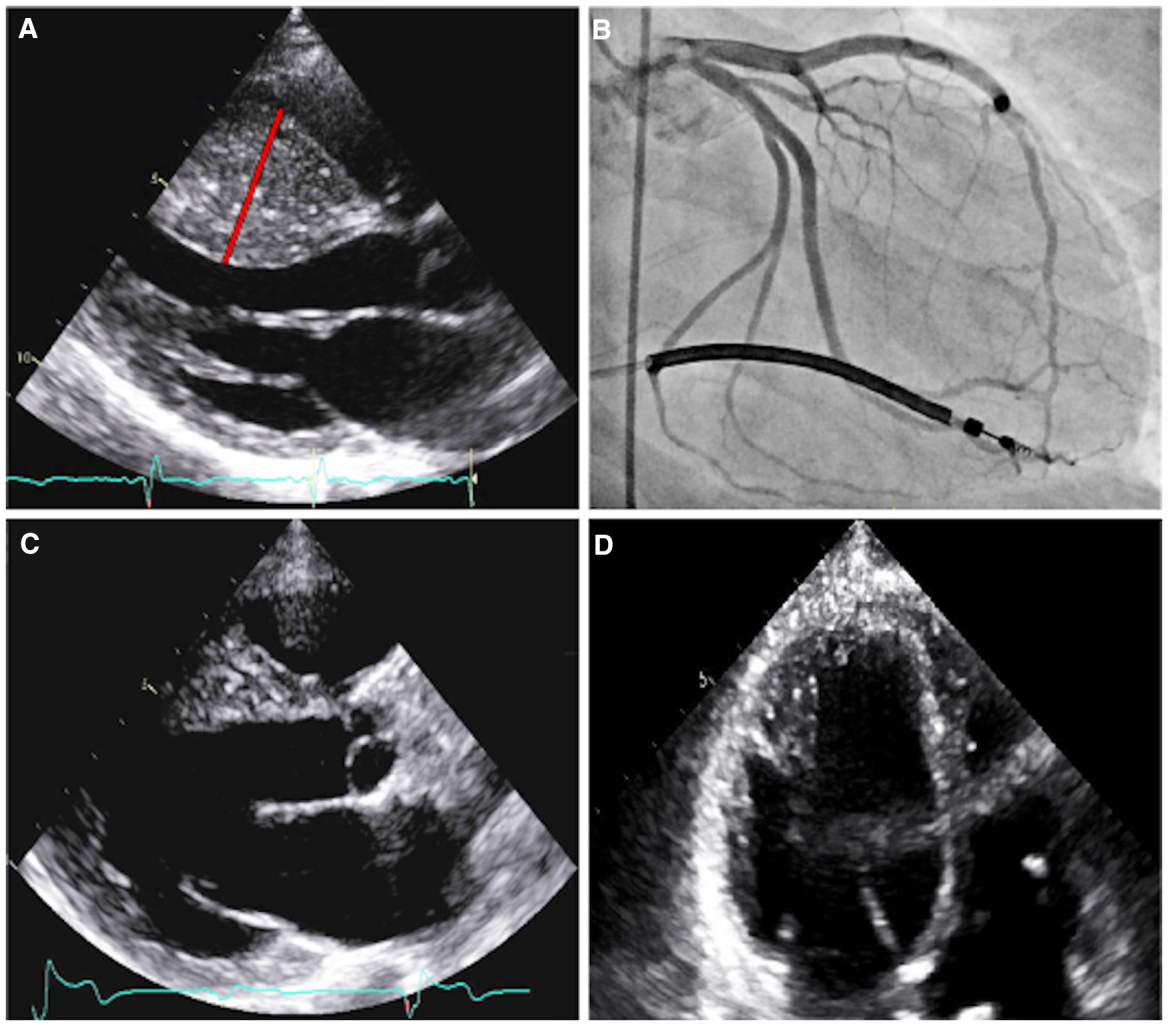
Imaging of patient A. Parasternal long axis echocardiogram prior to septal myectomy (A). The interventricular septum has reverse curve morphology, with a septal thickness measurement of 33 mm (red line) during diastole and a patent left ventricular outflow tract. Right anterior oblique caudal angiogram with prominent myocardial bridging of the large first and second septal perforators (B). Parasternal long axis echocardiogram, status-post septal myectomy (C). Four-chamber echocardiogram, with increased apical cavity size following septal myectomy (D).
